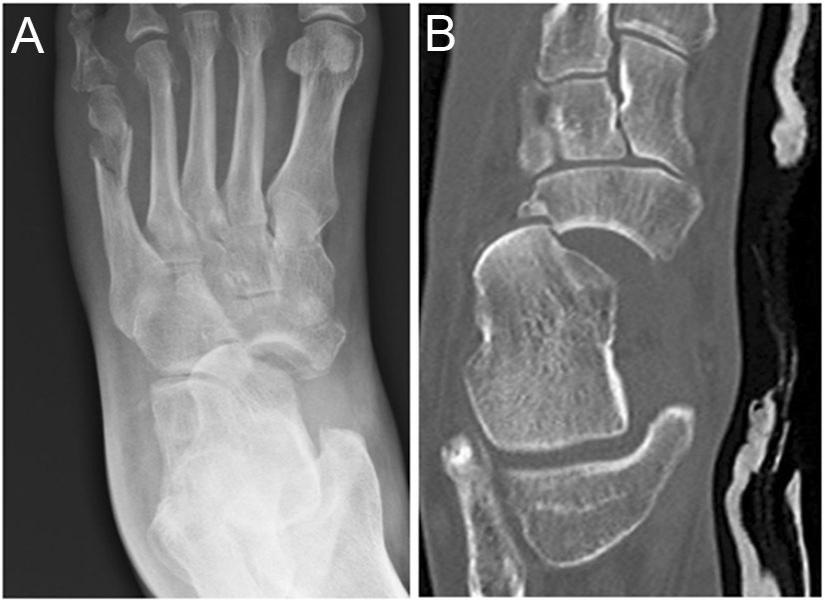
Chopart TNJ fracture-dislocation example. Anteroposterior radiograph (A) and axial CT (B) of a talar head dislocation with medial talar head and lateral navicular impaction injury
